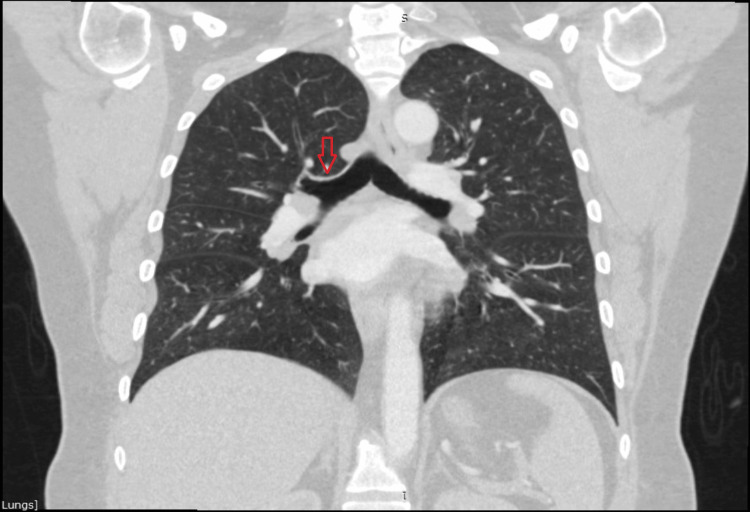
Follow-up CT scan Arrow pointing towards the previous tumor location.
